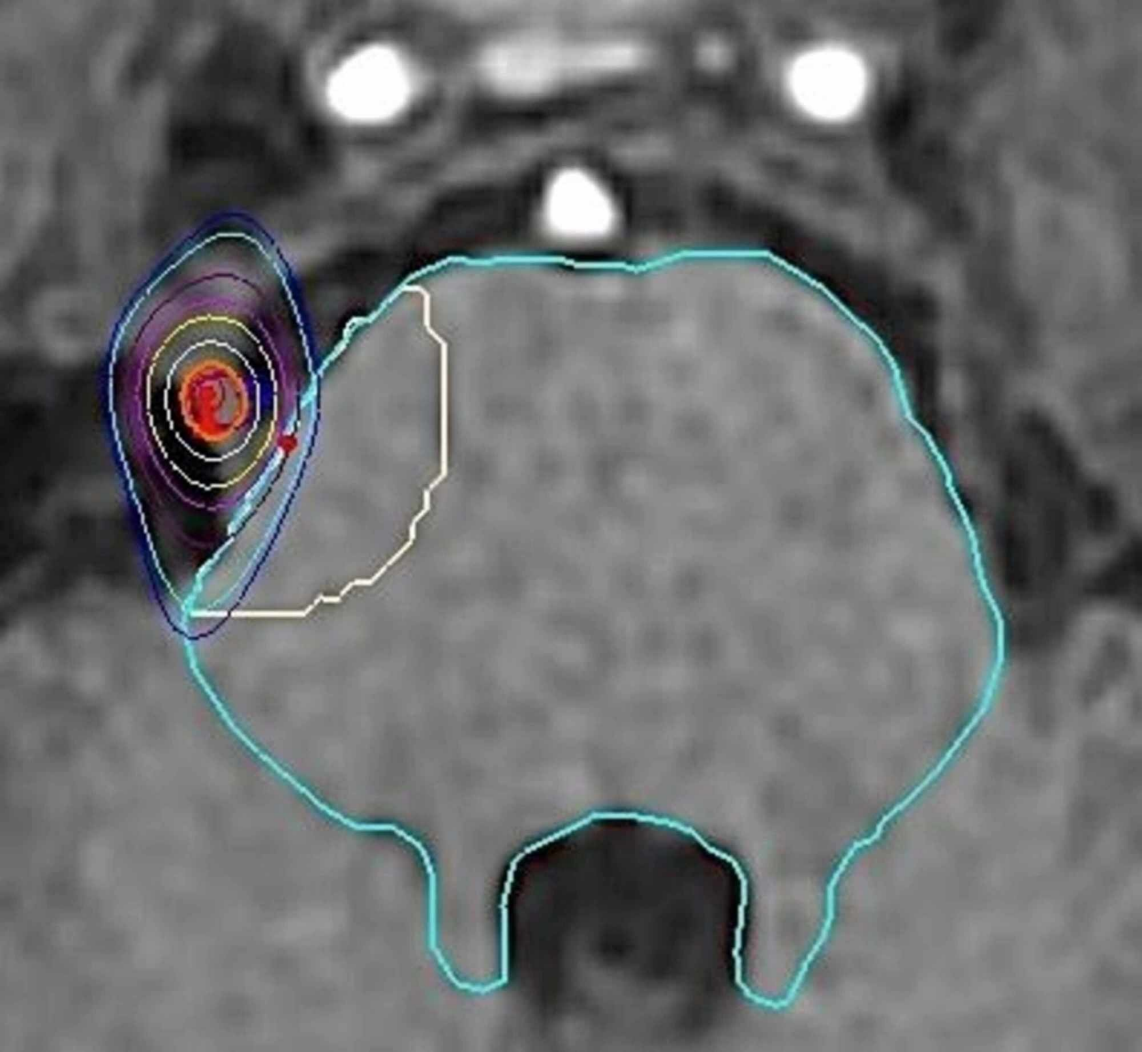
CyberKnife SRS treatment (MRI image) SRS: stereotactic radiosurgery; MRI: magnetic resonance imaging CyberKnife (Accuray Incorporated, Sunnyvale, California)
